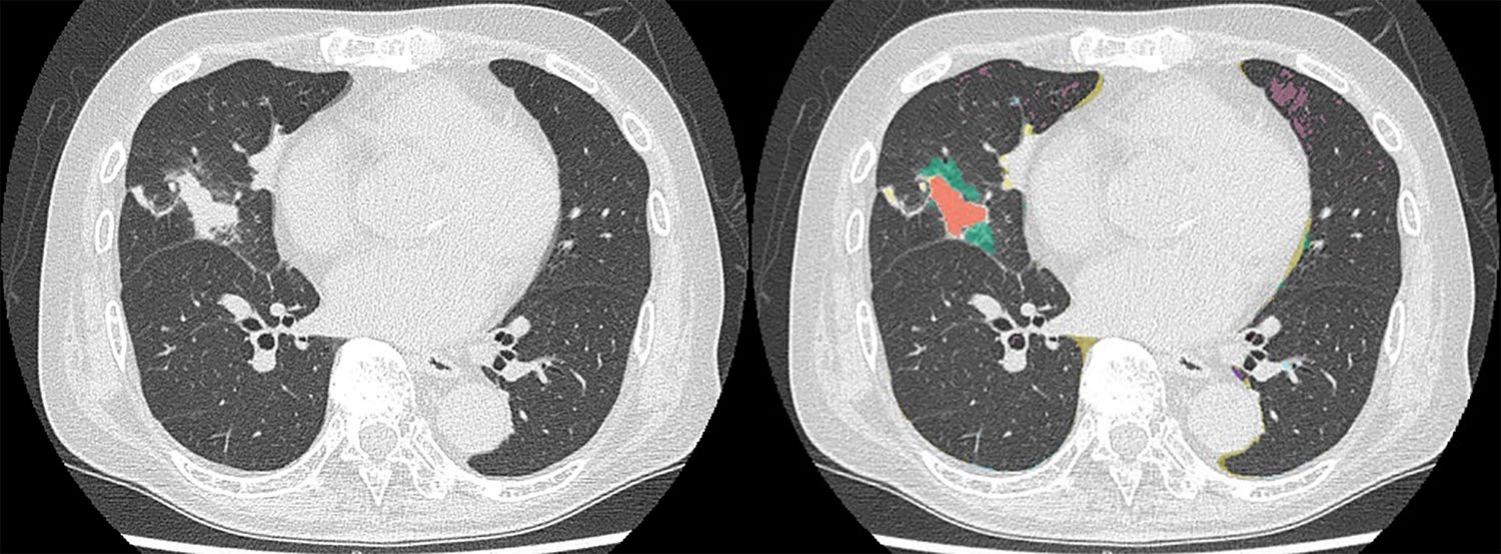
74-year-old male patient with suspected COVID-19 pneumonia and diagnosed as negative on RT-PCR. (L to R: thin-section CT to CAST result) On thin-section CT, consolidation with ground-glass opacities was observed in the right middle lobe, and emphysema in the lingula segment. CAST shows consolidation and ground-glass opacities as pale beige and green in the right middle lobe and emphysema as purple. The PCR test also identified this case as “negative”. All chest radiologists and the CAST software assessed this case as “atypical appearance” and “negative case”, so that it was judged to be true-negative
